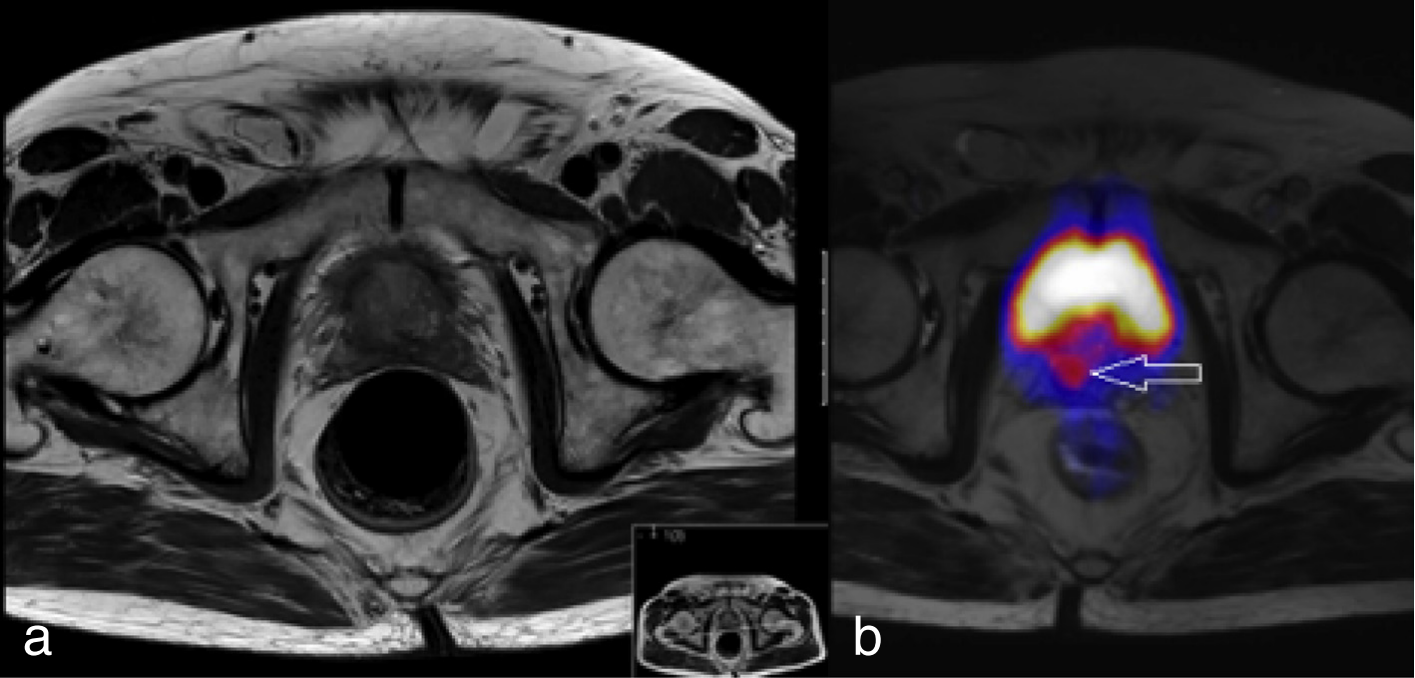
Up-staging due to extra capsular spread by ^68^Ga-PSMA-PET. Questionable extra capsular spread on MRI (a). In ^68^Ga-PSMA-PET imaging (b) is strong tracer uptake in the bladder and dorsal, as indicated by the arrow, in the extra capsular region, suggesting a strong possibility of its involvement
